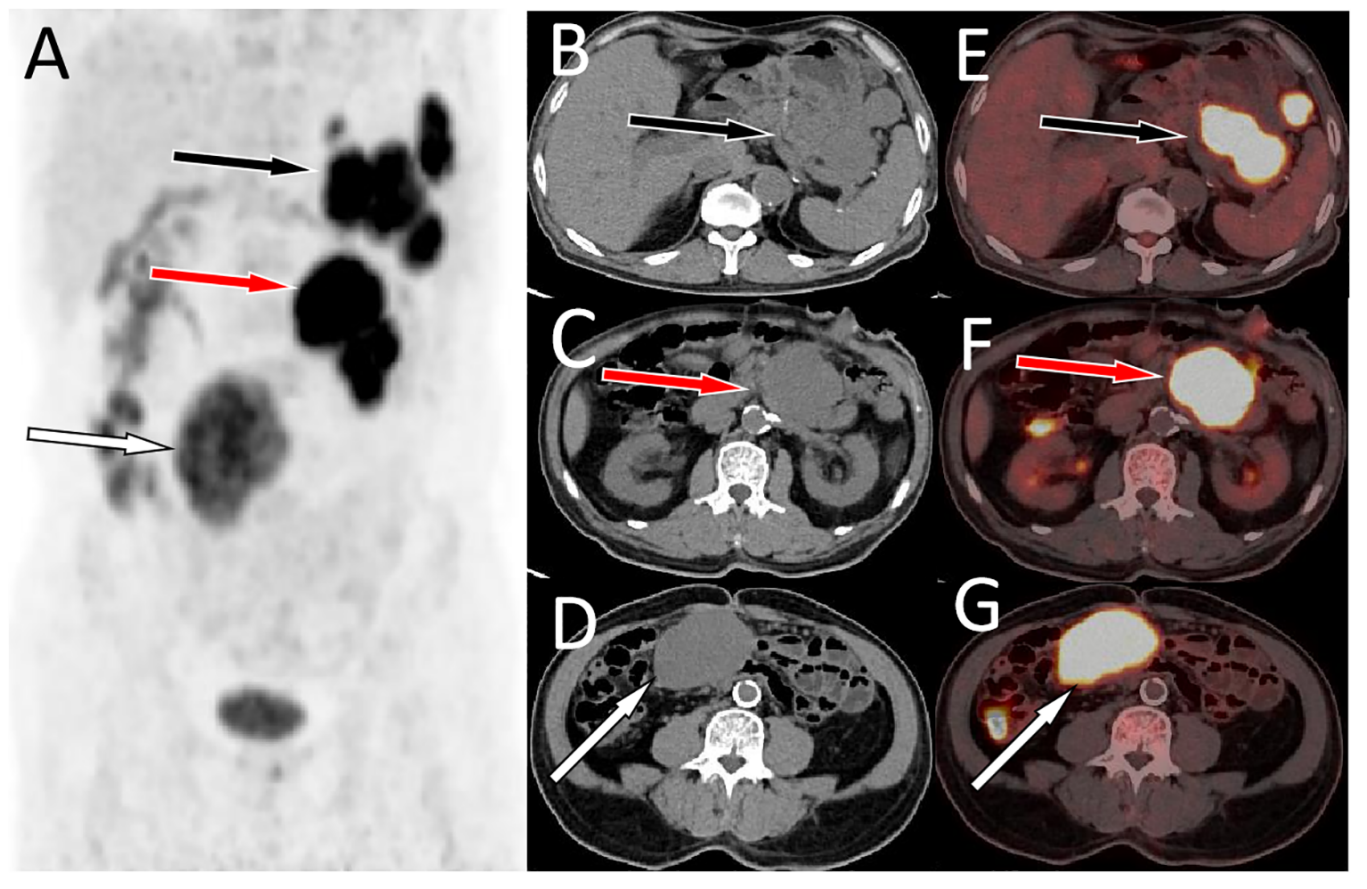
A delayed PET/CT scan at 180 min (A, MIP) was acquired, axial CT (B-D) and PET/CT fusion (E-G) showed a further increase in ^18^F-FDG radioactivity in the abovementioned lumps, with SUVmax of 16.0, 17.1, and 16.7 from top to bottom, respectively. Furthermore, the location of the lowest mass shifted from the initial left side of the spine to the right side of the spine (G, I, white arrows).Notes: MIP, maximum intensity projection; CT, computed tomography; PET, positron emission tomography; ^18^F-FDG, ^18^F-labeled fluoro-2-deoxyglucose; SUVmax, maximum standard uptake value.
